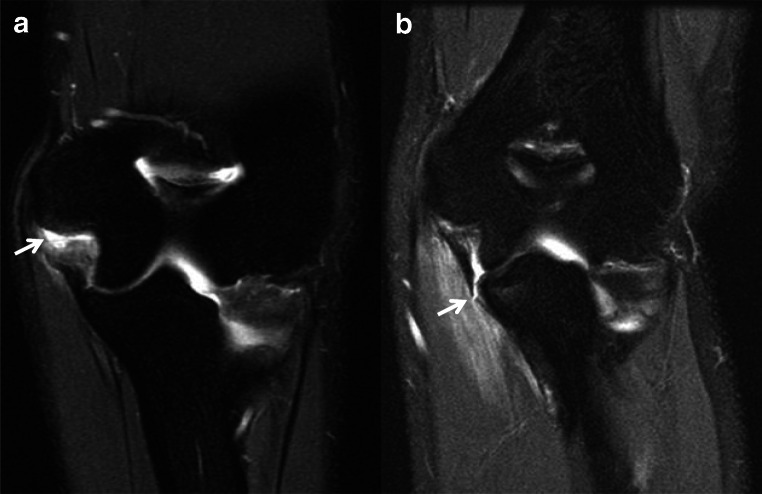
Coronal T2 MR images of the elbow in different patients demonstrate proximal (a, arrow) and distal (b, arrow) full thickness tears of the UCL
